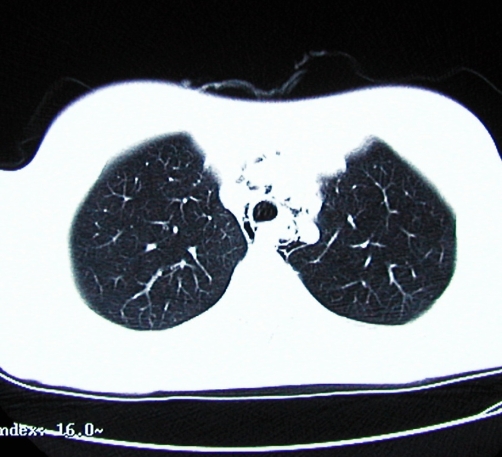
CT showing pneumomediastinum.
